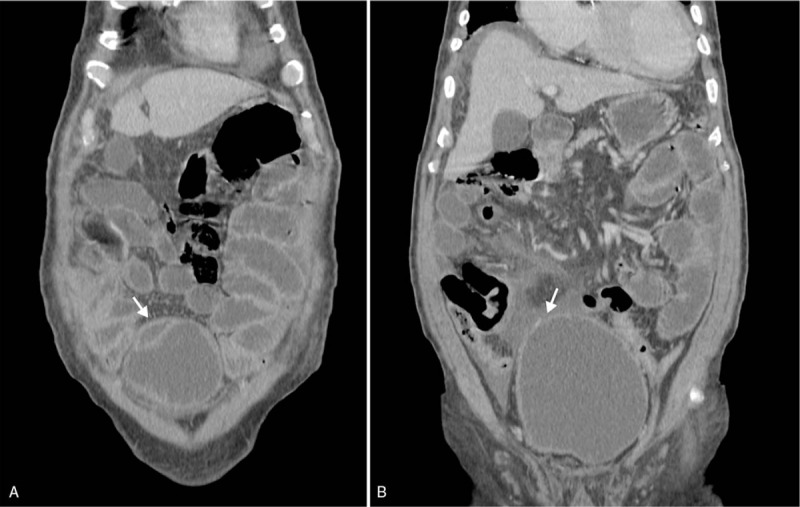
Coronal view of abdominal computed tomography. A. The black arrow indicates suspected infected diverticulum. B. The black arrow shows small defect in the dome of the bladder diverticulum. Small amounts of intraperitoneal fluid were observed.
